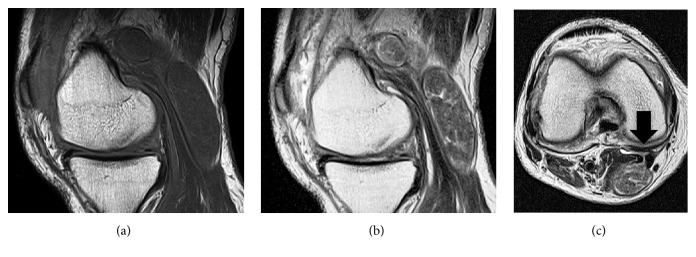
Preoperative sagittal T1-weighted (a) and T2-weighted (b) and axial T2-weighted (c) magnetic resonance images. A cyst was detected with low signal intensity on T1-weighted images and heterogeneous intermediate signal intensity on T2-weighted images. A connecting path was seen between the cyst and the subgastrocnemius bursa (arrow), compatible with the characteristics of popliteal cyst.
